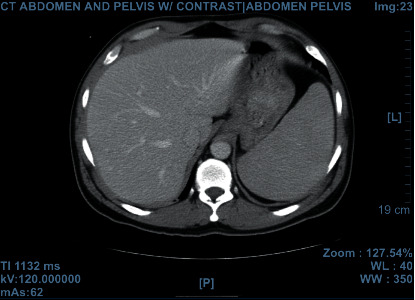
CT scan of abdomen and pelvis with contrast. Diagnosis of splenomegaly, 20 cm. Inferior peri-splenic hemorrhage, three grade laceration.
